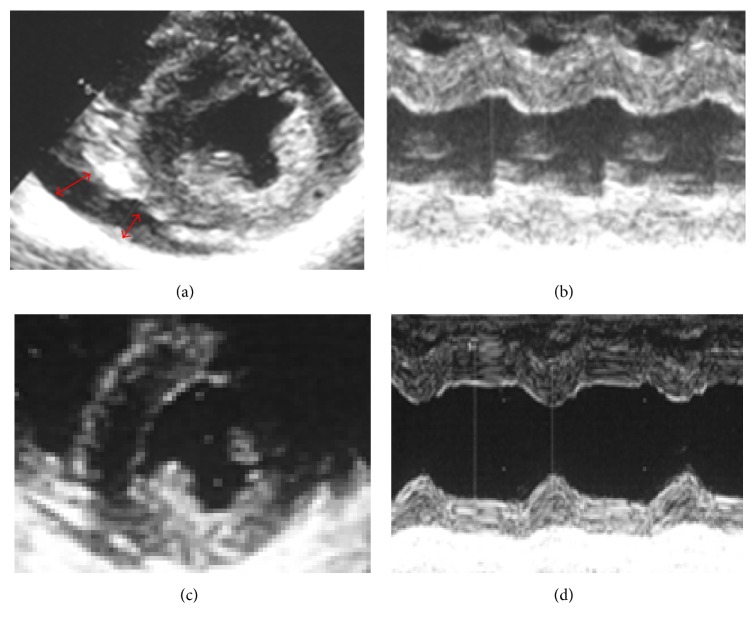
(a, b) Parasternal echocardiography at the onset of heart failure. (a) Short axis view showing pericardiac effusion (red arrow) and all-round ventricular hypertrophy. (b) Motion (M) mode through the left ventricle. The LVDd was 35 mm, left ventricular posterior wall systole (LVDs) 27.6 mm, LVPWD 12.6 mm (196% of normal), and interventricular septal end diastole (IVSd) 13.3 mm (211% of normal). Also, the calculated LVEF was 55%. These findings suggest that diastolic dysfunction due to biventricular hypertrophy resulted in heart failure. (c, d) Parasternal echocardiography 7 days after the initiation of steroid pulse therapy. The LVDd was 40 mm, left ventricular posterior wall systole (LVDs) 23 mm, LVPWD 8.7 mm (133% of normal), and interventricular septal end diastole (IVSd) 10 mm (158% of normal). Also, the calculated LVEF was 80%. Biventricular hypertrophy was improving although still residual, and cardiac contractility was significantly improved.
